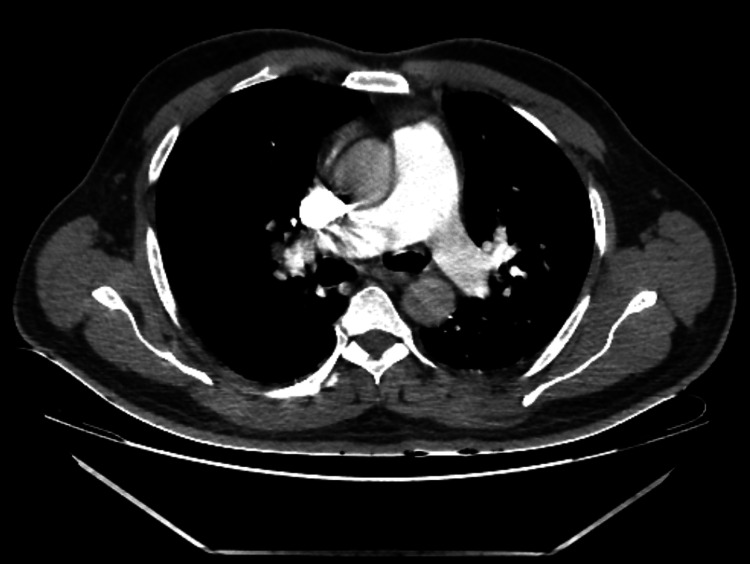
CT angiogram of the pulmonary arteries showing no evidence of pulmonary embolism.
